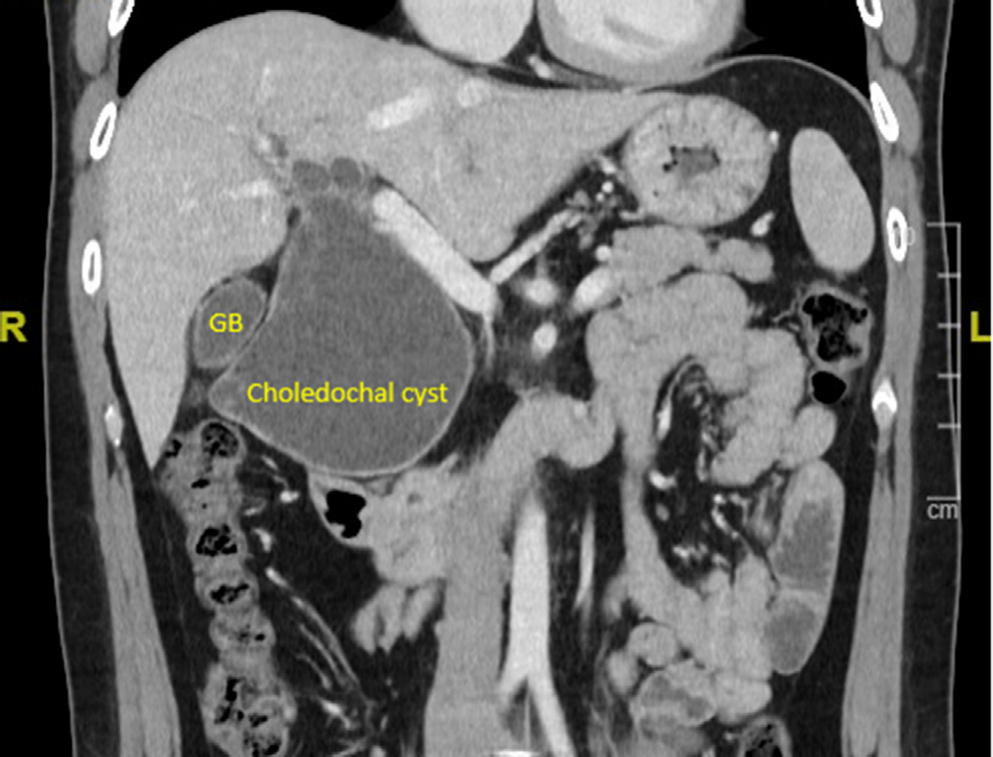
Computed tomography of the abdomen and pelvis showed a large choledochal cyst and normal GB. GB, gall bladder.
